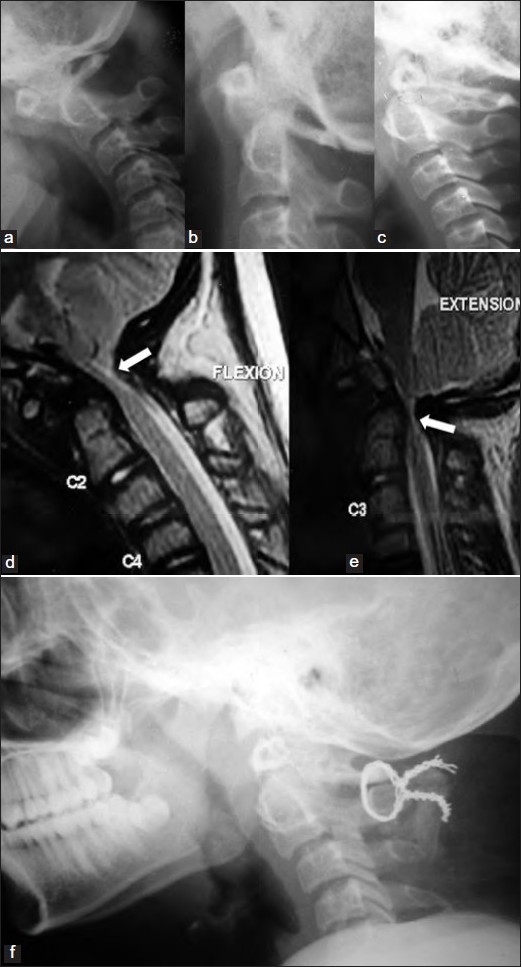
Plain lateral radiographs of craniovertebral junction in flexion (a), neutral position (b) and extension (c) of neck showing cervicomedullary compression in both flexion and extension. T2-weighted sagittal MR images in flexion (d) extension (e) showing cervicomedullary compression (arrows) in both flexion and extension. Postoperative radiograph (f) showing C1-2 sublaminar fusion with mild OO retrolisthesis
